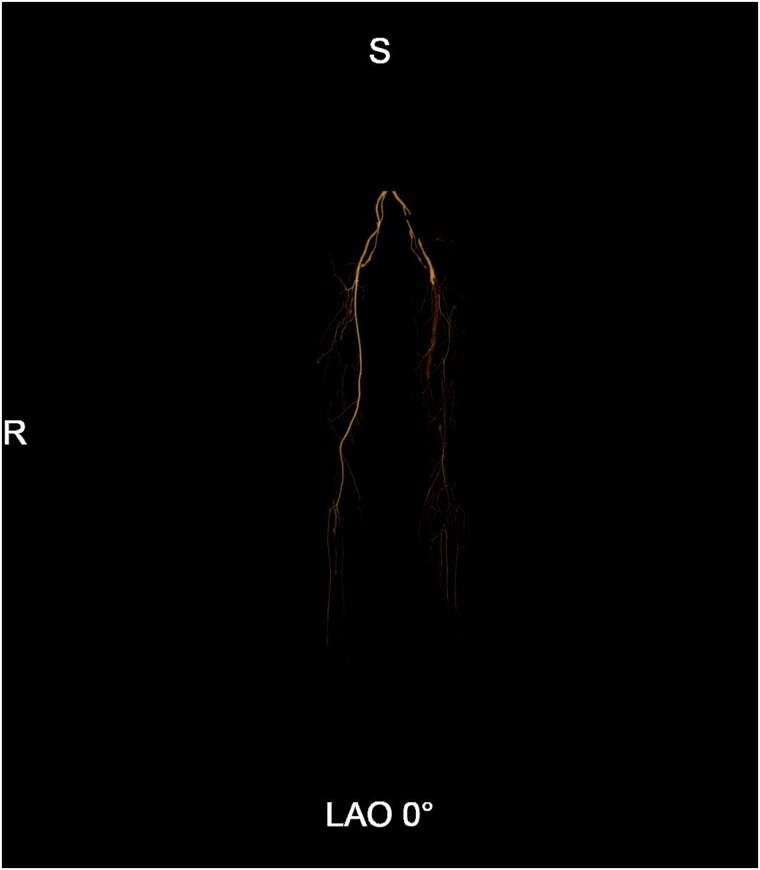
CT angiography revealed occlusion of the left femoral and popliteal arteries, with distal vessels visualized intermittently.
